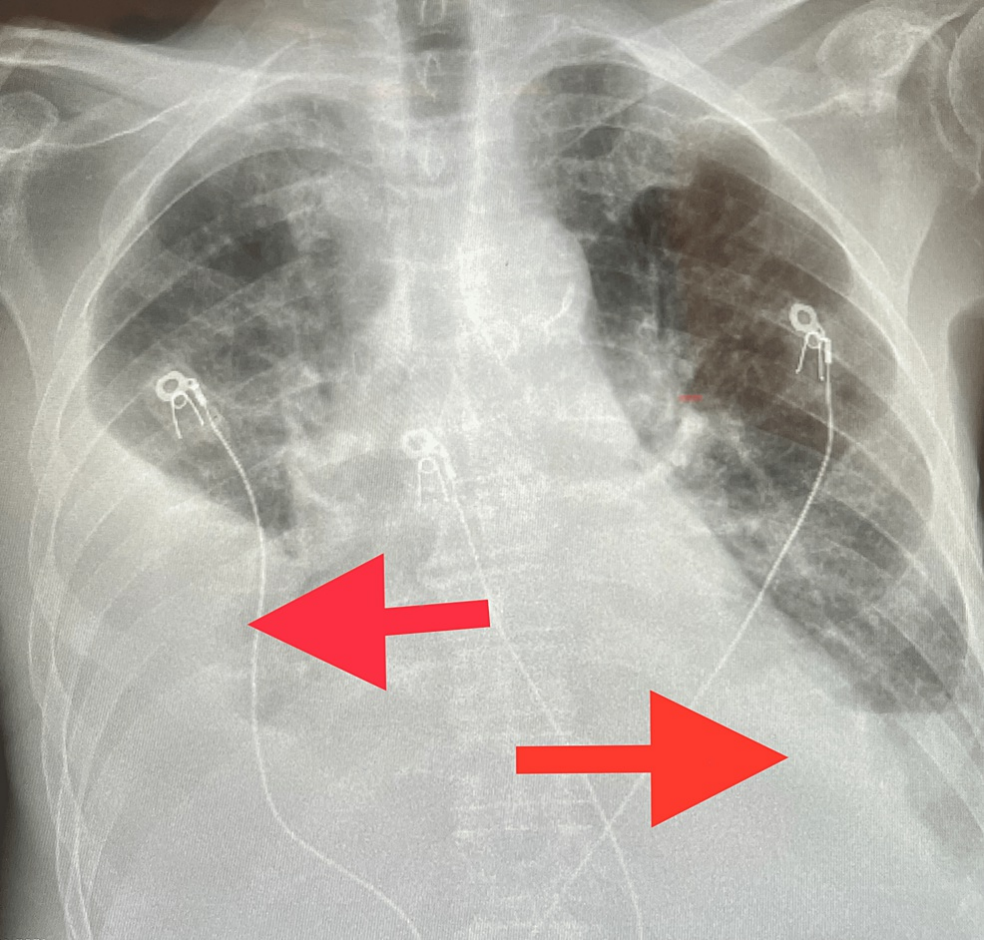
Chest X-ray showing large right-sided and moderate left-sided pleural effusion (red arrows), with bibasilar consolidation
